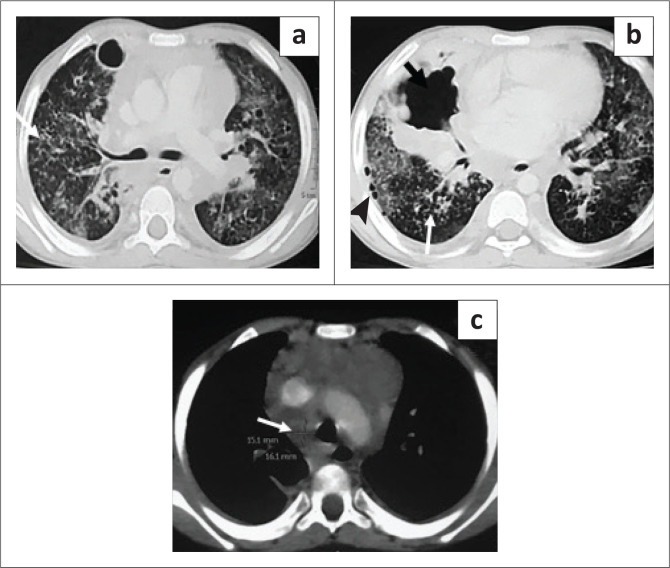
Axial CT performed 2 months prior, (a, b) showed cavitation in the right middle lobe (black arrow) with ground glass opacities in both lungs, a few tiny cysts in the right lung (arrowhead) and multiple bilateral nodules (white arrow). Axial mediastinal window (c) shows enlarged right paratracheal lymph nodes (arrow).
